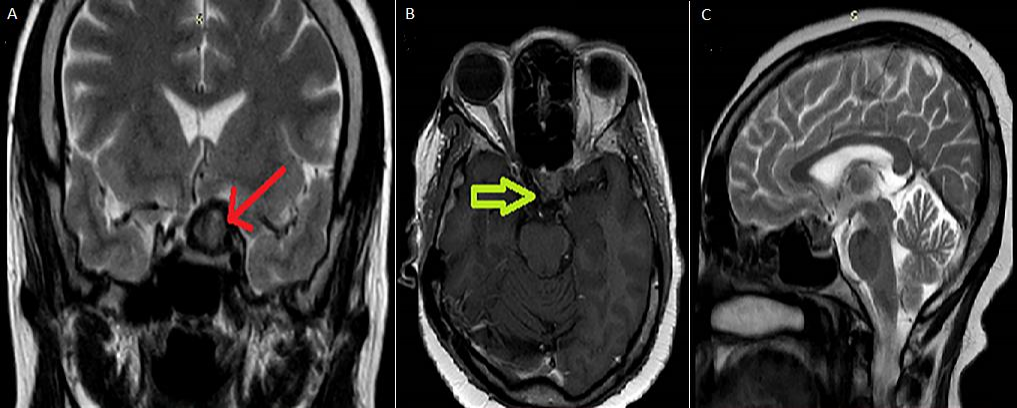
A,B,C) T2 coronal view, axial view of T1 contrast and T2 sagittal view respectively of the patient´s brain MRI scan demonstrating a sellar space-occupying lesion with moderate contrast enhancement (green block arrow in B) and a hypointense periphery (red line arrow in A)
